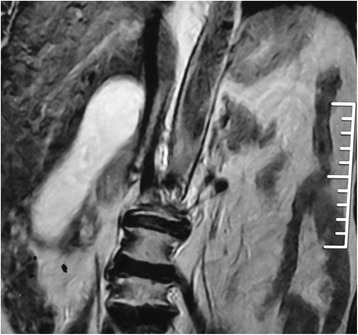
MRI showing mucocele of gall bladder (coronal plane)
